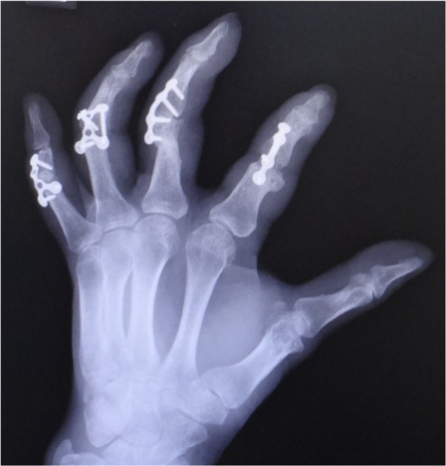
An X-ray image after arthrodesis with micro-plates at an angle of 45° of the PIP joints
